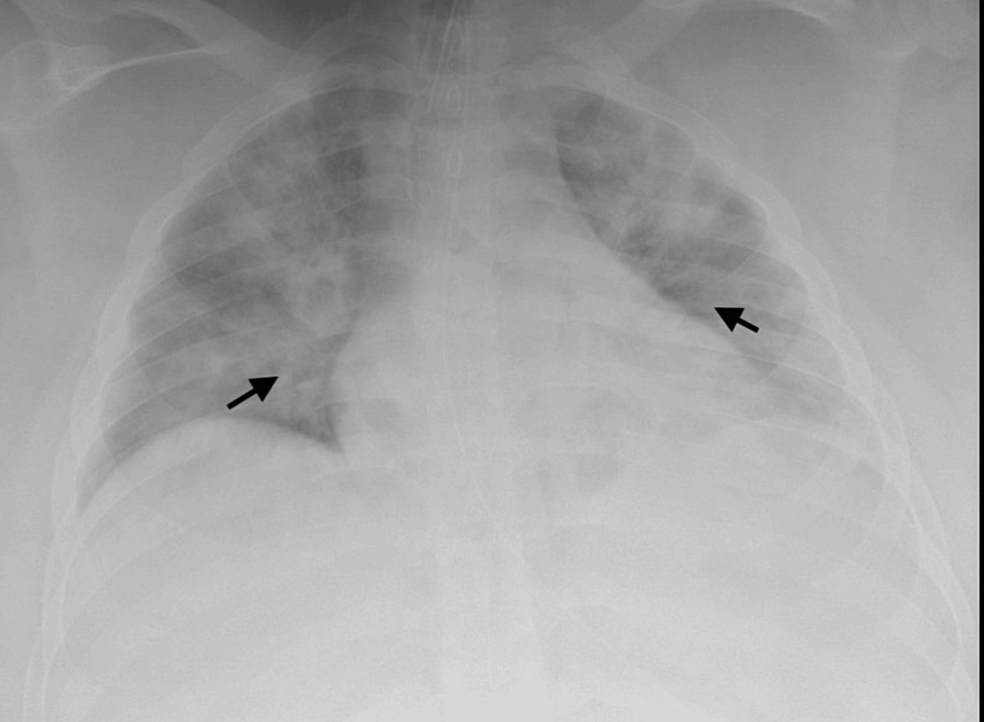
Case 2 chest X-ray Arrows indicating bilateral pulmonary infiltrates
